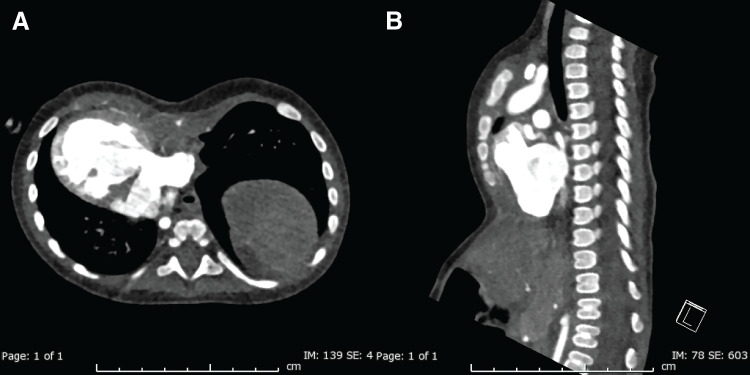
Preoperative contrast-enhanced CT. (A) Axial view demonstrating severe pectus excavatum with a Haller index of 3.5 and hypertrophic retrosternal soft tissue. (B) Sagittal view confirming the depth of sternal depression and reduced mediastinal space.
